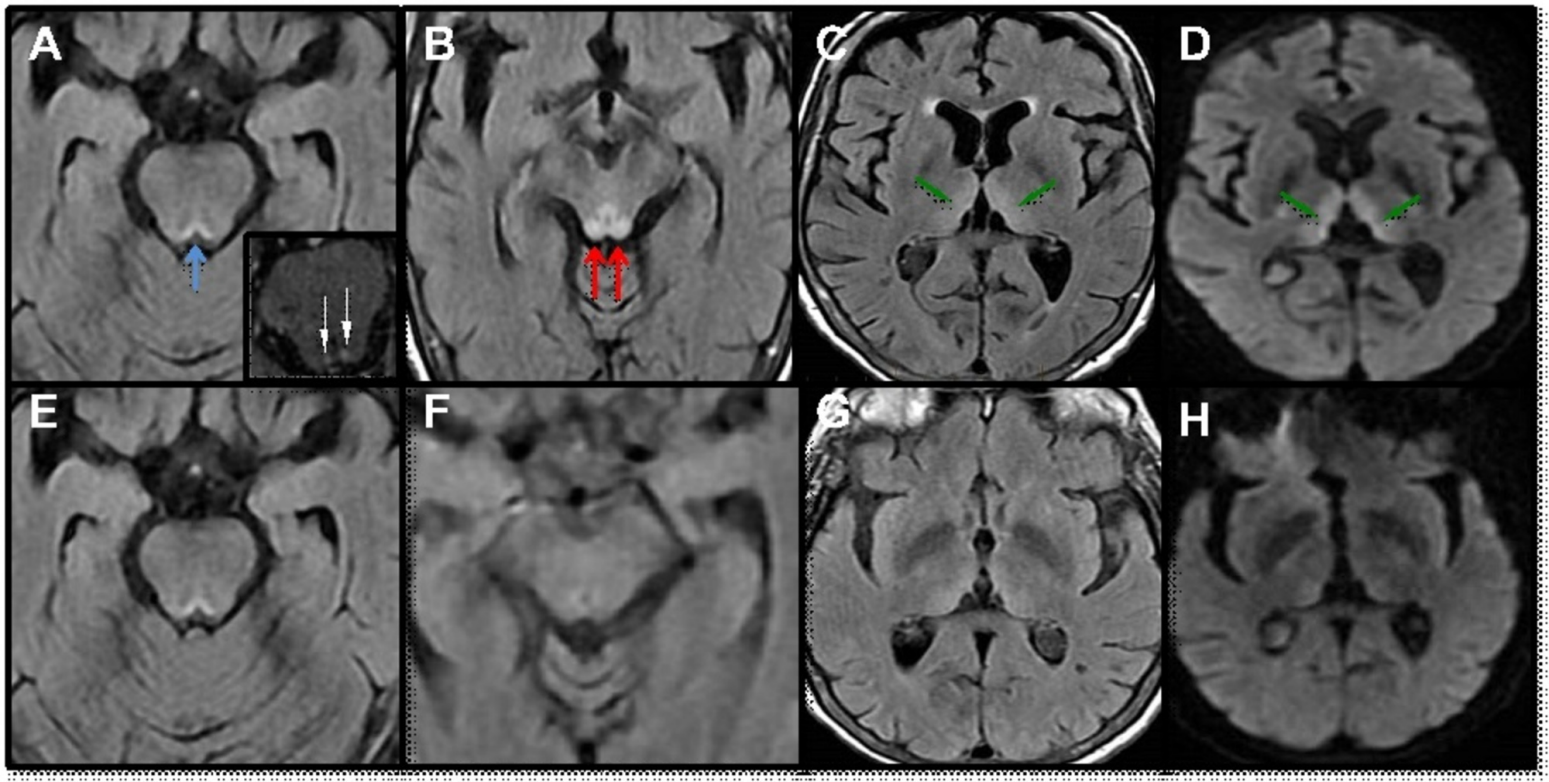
Changes on MRI with Wernicke's encephalopathy MRI brain with contrast showing T2 FLAIR signal hyperintensity involving the inferior tectal plate (A, blue arrow) with enhancement (A, inset) as well as the periaqueductal gray matter within the dorsal midbrain (B, red arrow). There is also involvement of the the medial thalami bilaterally (green arrow) on T2 FLAIR (C) as well as on DWI (D). Patient was treated with high dose intravenous thiamine with repeat MRI brain, one week later showing a significant improvement (E, F, G and H) MRI: magnetic resonance imaging, DWI: diffusion-weighted imaging
